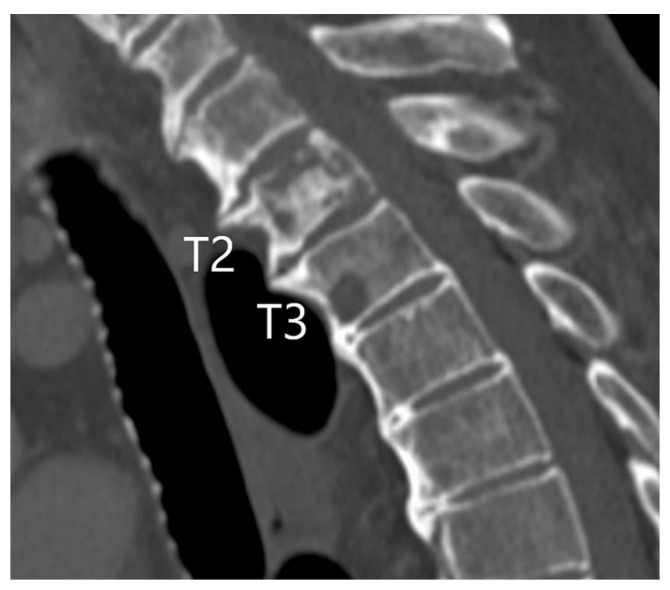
M1c1 category: A sagittal reformatted CT image (bone window) showing lytic metastasis in the T3 vertebral body, with mixed lytic and sclerotic metastatic lesions in the T2 vertebral body. No other distant metastases were evident.
